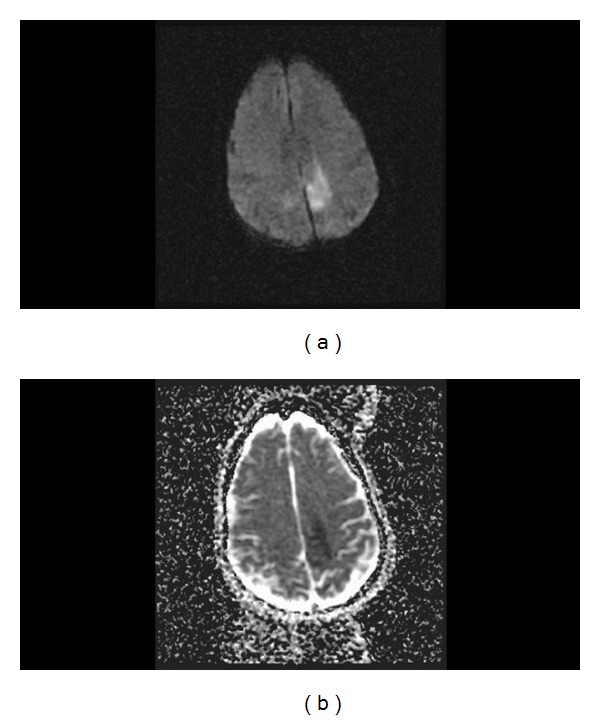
Axial diffusion-weighted (a) and ADC (b) MR images of the brain on postoperative day 5 revealing focal restricted diffusion and low ADC signal in the left posterior frontoparietal area consistent with acute infarction.
